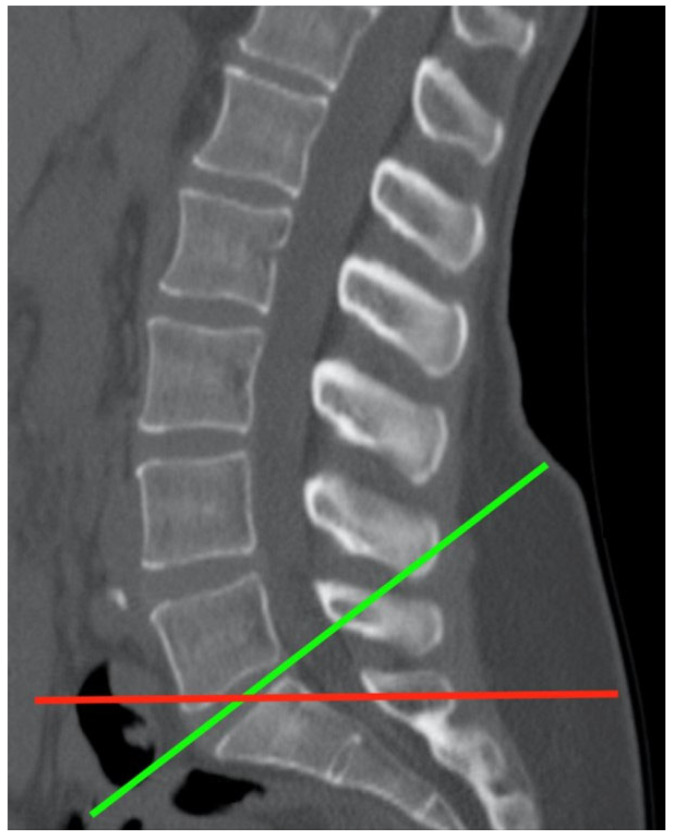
A sagittal cross-section of a lumbar spine CT scan: the red line indicates the volume slice and the green indicates the reconstructed slice.
